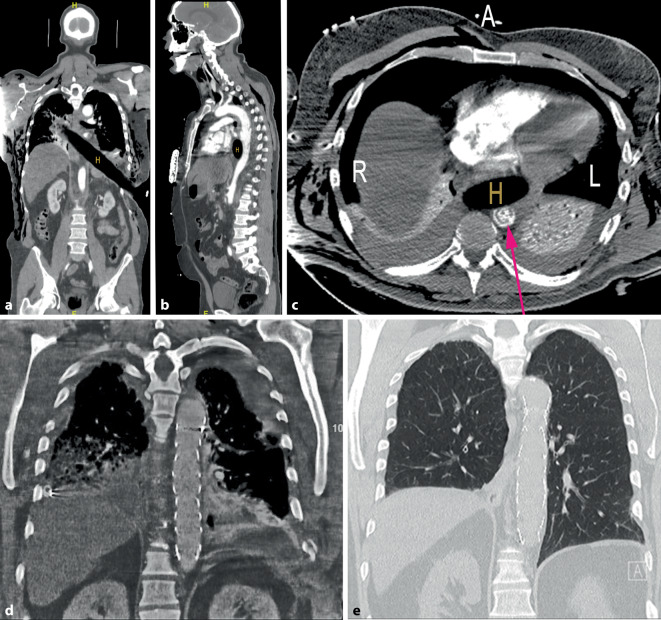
a–e Computertomographische Darstellungen. a–c zeigen am Unfalltag die Lagebeziehung zwischen dem Holzstiel (*H*) und den Organen. Die Aorta ist durch den anliegenden Fremdkörper verletzt und verlagert. d zeigt 10 Tage nach Unfall die Aorta mit Stent. e CT-Thorax 7 Jahre später. Das Lungenparenchym hat sich restrukturiert. Zwerchfellhochstand rechts und fehlende Milz links. Keine Diaphragma-Hernie. *R* rechte und *L* linke Thoraxseite, *A* anterior, *H* Holzpfahl. *Pfeil* Aorta descendens mit Dissektionsmembran
